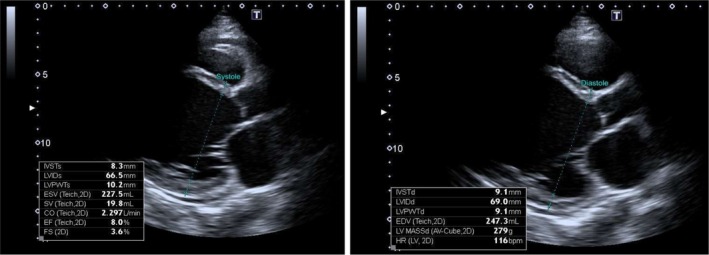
Echocardiogram showing increased left ventricular diameter during systole and diastole.
